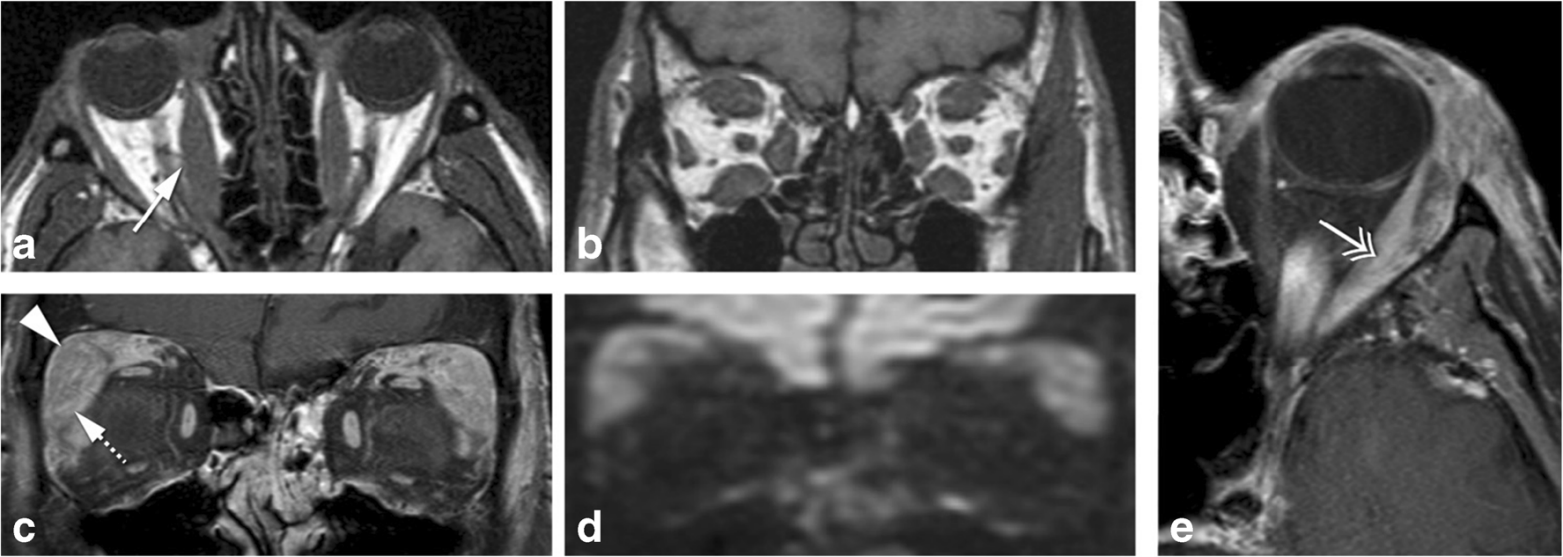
a, b 40-year-old female with thyroid-associated orbitopathy. Axial (a) and coronal T1-WI (b): bilateral and symmetric enlargement of the inferior, medial and complex levator palpebrae-superior rectus muscles. Notice fusiform configuration of the muscles because sparing of the tendon (arrow) and no surrounding infiltration of the fat. c–e 35-year-old male with IgG4-related disease. Coronals enhanced T1-WI with fat signal suppression (c) and DWI (d) and axial enhanced T1-WI with fat signal suppression (e): bilateral involvement of the superolateral quadrants of the orbits, including bilateral enlargement of the lacrimal glands with involvement of some of the adjacent muscles. Involvement of both the orbital (arrowhead) and palpebral (dashed arrow) lobes of the lacrimal gland. Fusiform configuration (double-headed arrow) of the muscle. No diffusion restriction favoring an inflammatory process
